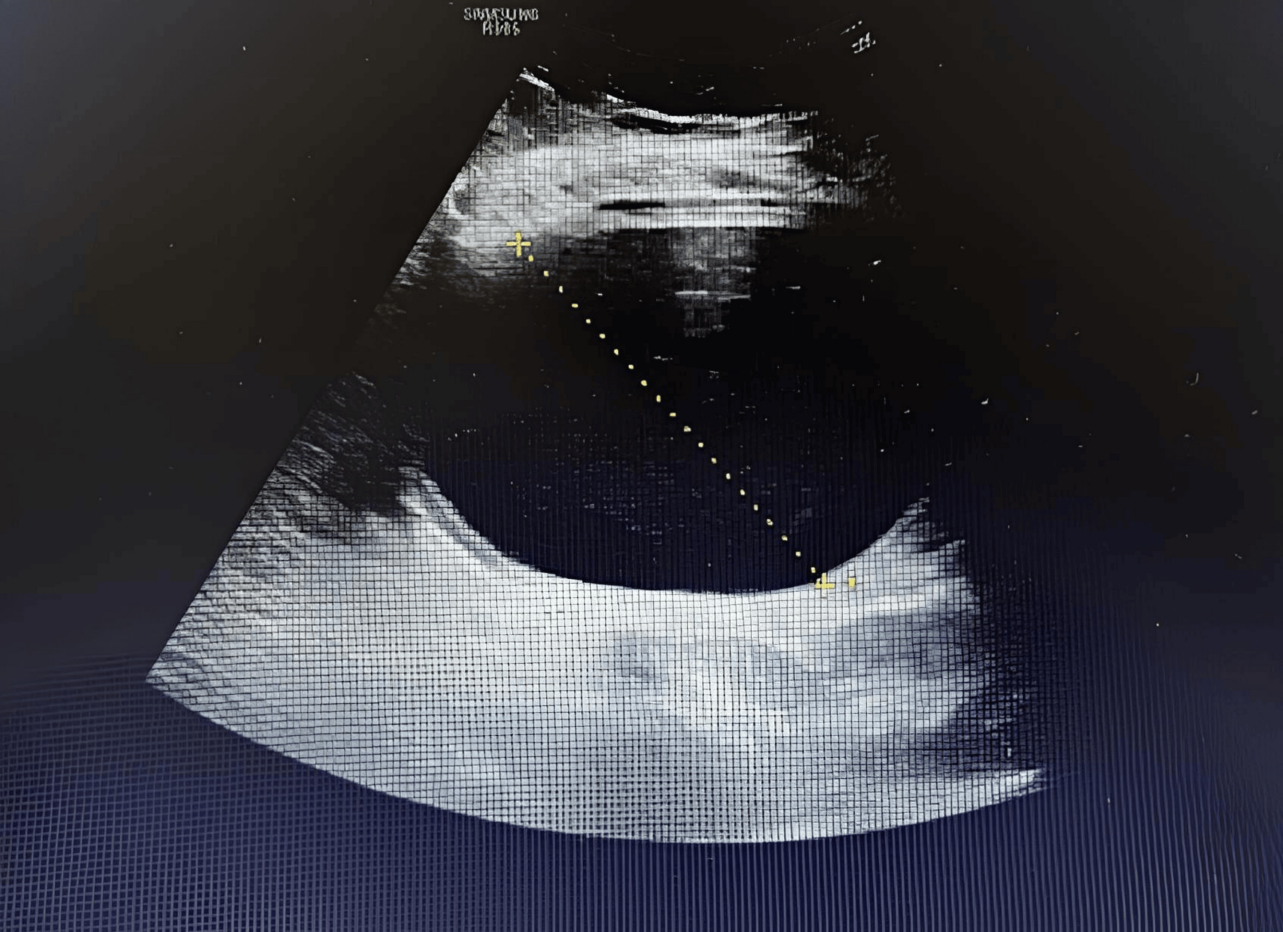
Abdominal ultrasound showing a fluid-filled gastric balloon in the antrum.
